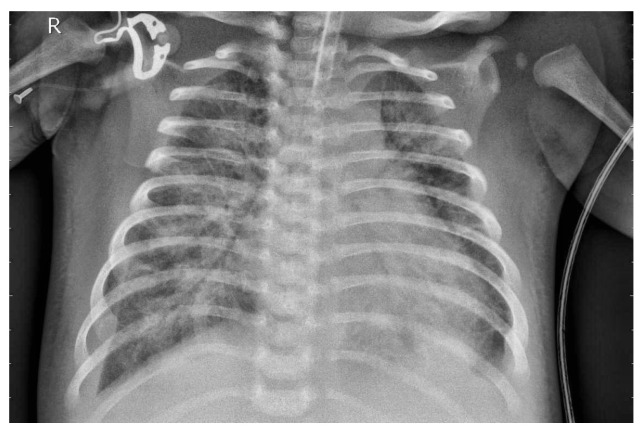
X-ray image after preprocessing.
